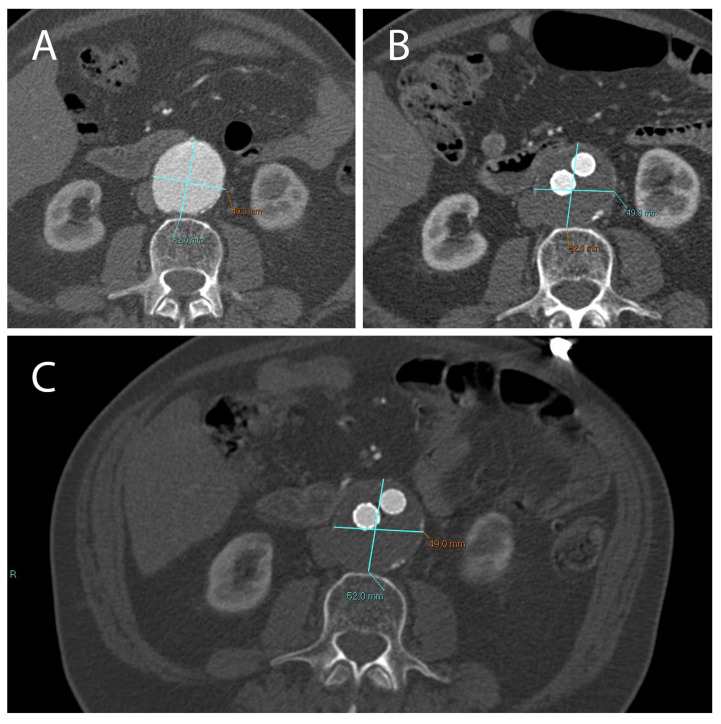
A not correctly excluded AAA treated with EVAR, with the presence of an endoleak. Treatment did not result in a reduction in aneurysm diameter compared to the pre-EVAR CTA (A). CT images obtained 1 month (B) and 12 months (C) after EVAR implantation.
